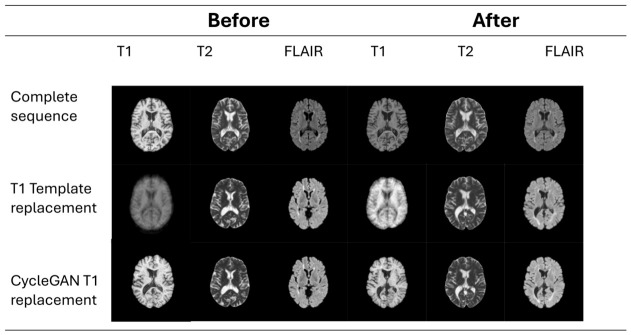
Example imaging co-registration schemes for subjects with or without ‘missing’ T1-weighted (T1) MRI. Top row shows a subject with all sequences obtained, so the images are co-registered to local T1 and then to the MNI T1 template. Middle row shows a subject without T1 acquired. It is first replaced by the T1 template generated in this study, which is then used to co-register local images to the MNI T1 template. Similarly, the bottom row also shows a subject without T1 acquired, while it is replaced by T1 images generated by the corresponding FLAIR using the cycle-consistent generative adversarial network (CycleGAN).
